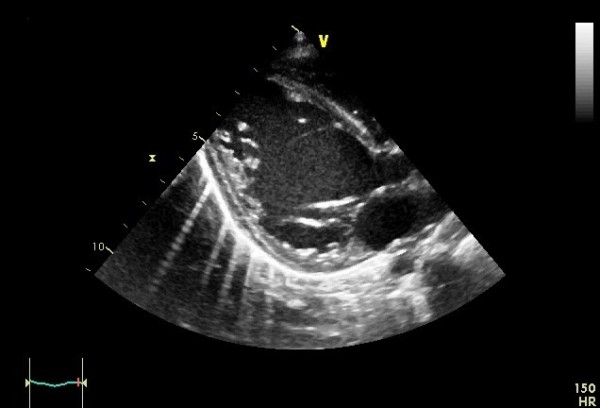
Transthoracic echocardiography. Dilated left ventricle.
